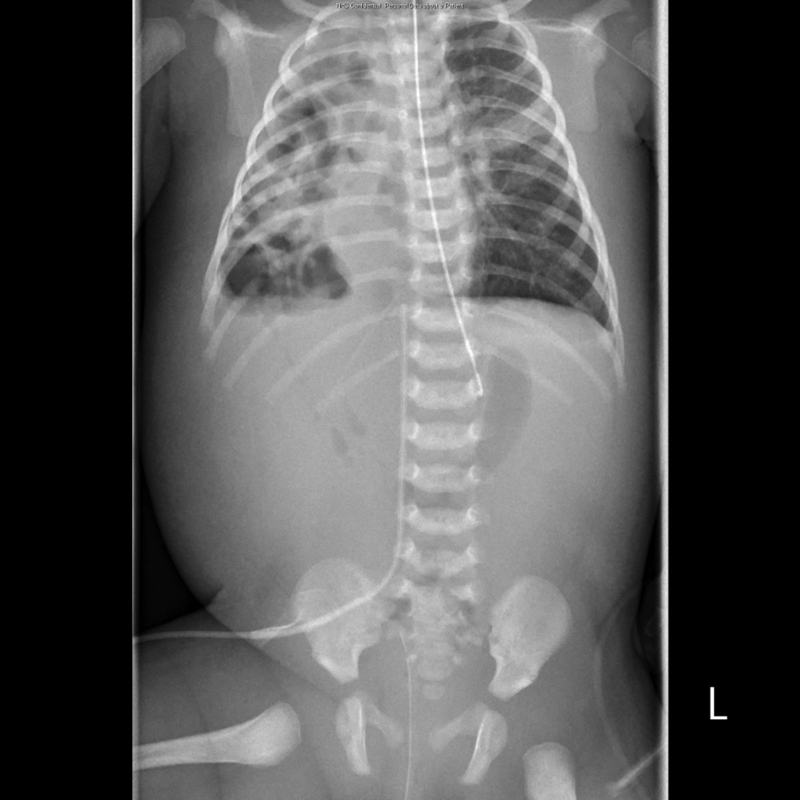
X-ray showing distended bowel loops in right hemithorax prior to emergency surgery in NICU. NICU, neonatal intensive care unit.
